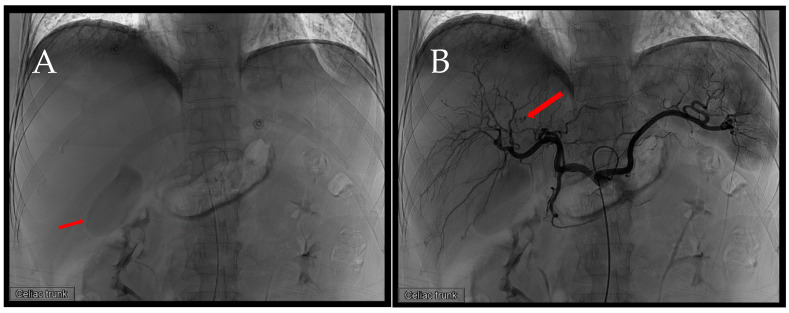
First TAE. After accessing the celiac trunk using a 4Fr. RH catheter, we performed a series of angiographies. (A) Prior to contrast injection, an evident hyperdense lesion (arrow) corresponding to the location identified in the CT scan is observed. The increased density is likely attributed to contrast extravasation during the preceding CT, thereby manifesting on this image. (B) A distinct extravasation (arrow) is visible, but its location does not align with the previously identified position in the CT scan.
